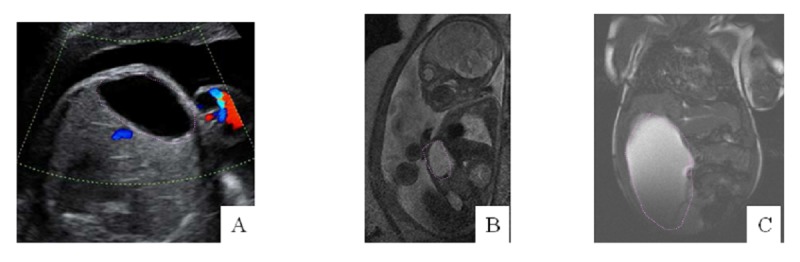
Figure 1: Patient 1 imaging: prenatal US (A) at 31 weeks of gestation with discovery of anechogenic lesion forward the liver, confirmed by MRI (B). Postnatal MRI (C) (at 6 weeks of age) attesting rapid cyst growing.
